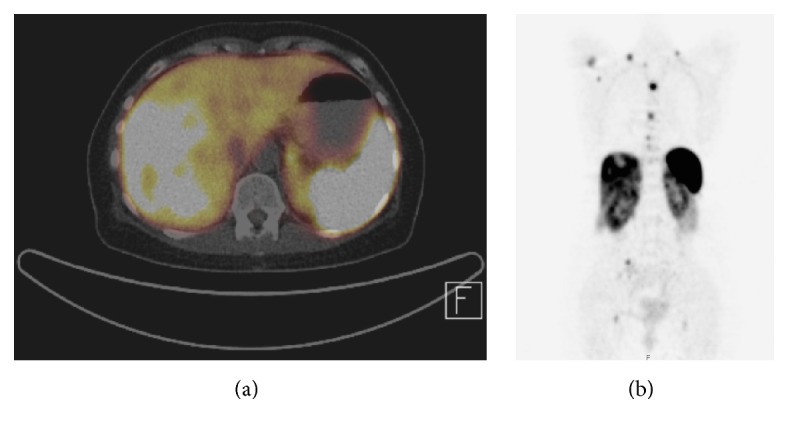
Somatostatin receptor imaging with CT-scan (PET-CT) (a) showing extensive liver metastasis in the right liver lobe in 12/2011 and (b) additionally showing hydronephrosis.
